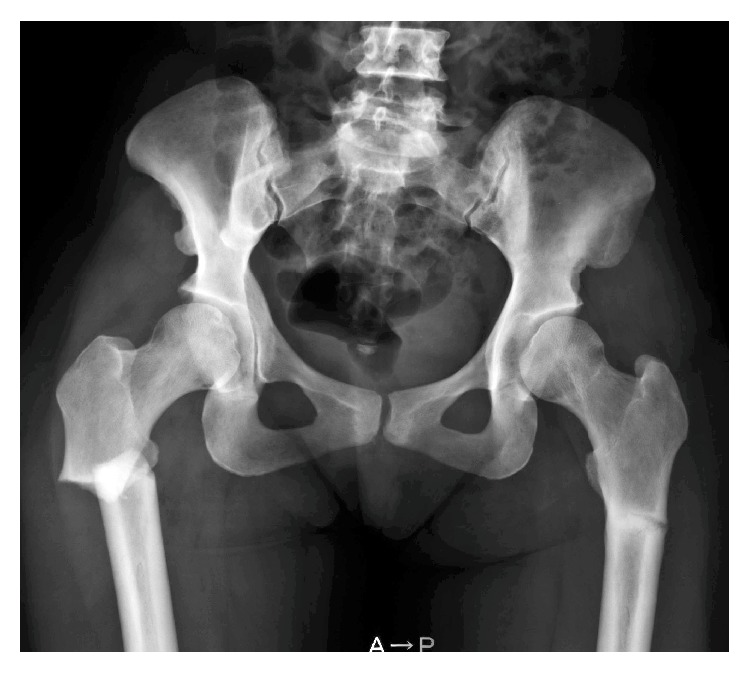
Radiograph showing subtrochanteric fracture of the right femur and a stress fracture in the left femur in the same region. Bones are sclerotic and medullary canal is visible.
